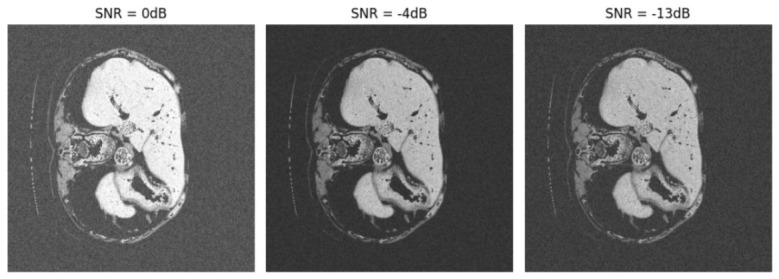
The noisy CT slice with different SNRs.
